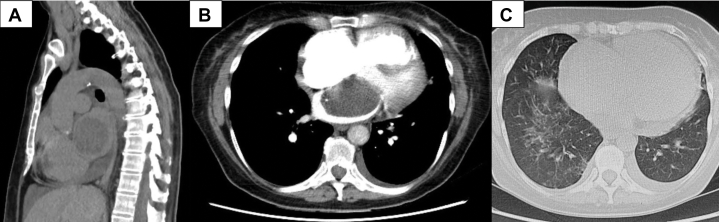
Computed Tomography Angiography (A and B) Computed tomography angiography showed a giant left atrial mass and light pericardial effusion. (C) Signs of heart failure were also observed.
